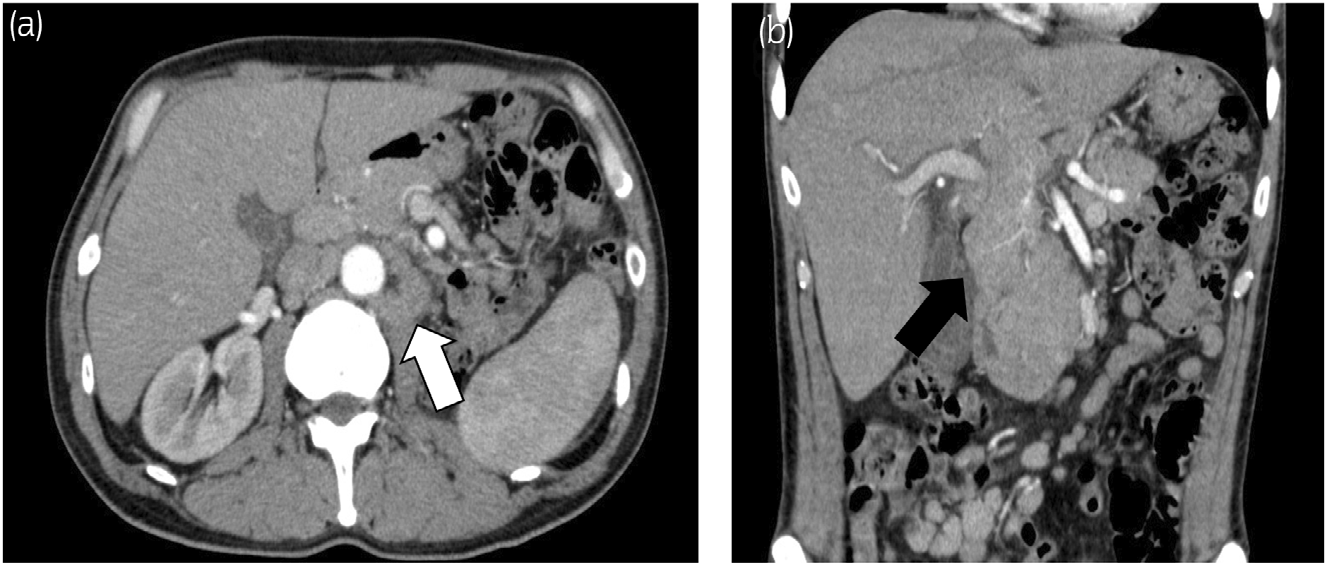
Abdominal computed tomography findings. An enlarged para‐aortic lymph node (white arrow) is visible; however, it did not significantly differ from before Pem treatment (a). The hilar and mesenteric lymph nodes (black arrow) are prominently enlarged (b).
